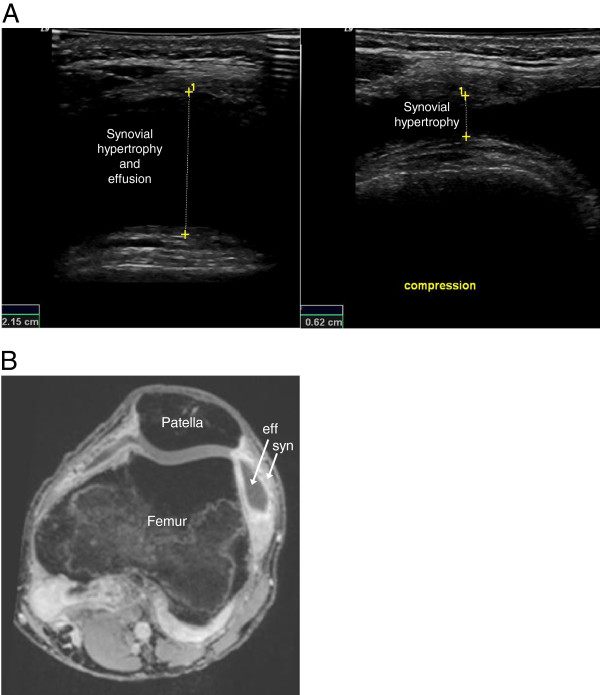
Imaging of the symptomatic and swollen knee of a 17-year-old boy with JIA. (A) Axial US scanning lateral to the patella. The left image shows an anechoic recess composed of synovial thickening and effusion, measured (1) before compression. In the right image, compression of the recess has transposed the effusion and enabled measurement (1) of the hypertrophic synovium. (B) A 3D T1 gradient echo VIBE MRI image of the same joint showing the enhanced hypertrophic synovial tissue (syn), and the effusion (eff), following intravenous injection of Gadolinium contrast.
